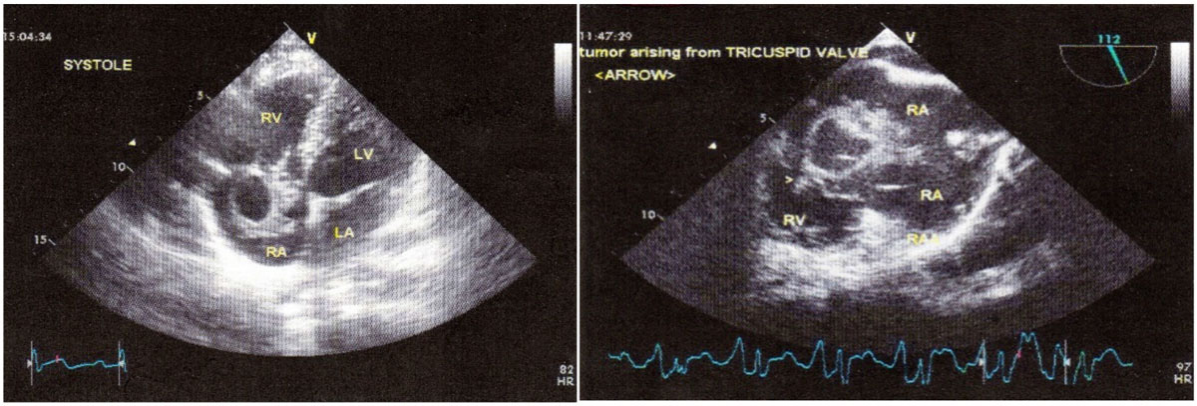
TTE-TEE Echocardiography: Right atrial mass.
